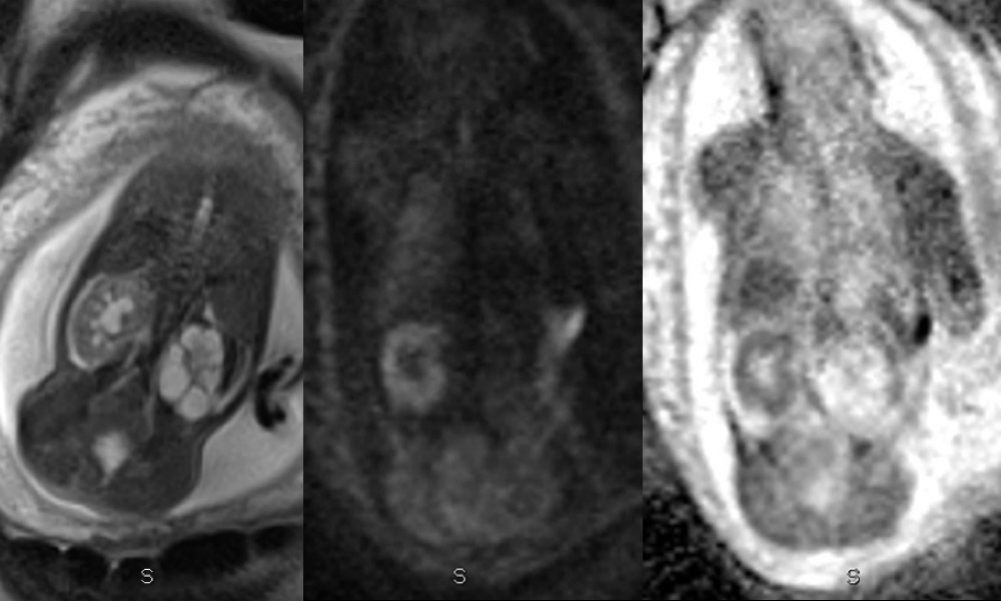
Coronal T2 HASTE sequence shows a right kidney with pelvicaliceal dilatation in a fetus at 28 weeks. The renal parenchyma on the corresponding DWI sequence returns high signal, with low values on the ADC map suggesting restriction of free water diffusion. In contrast, there is no corresponding high DWI signal returned from the left-sided multicystic dysplasic kidney, suggesting the absence of normal renal tissue.
